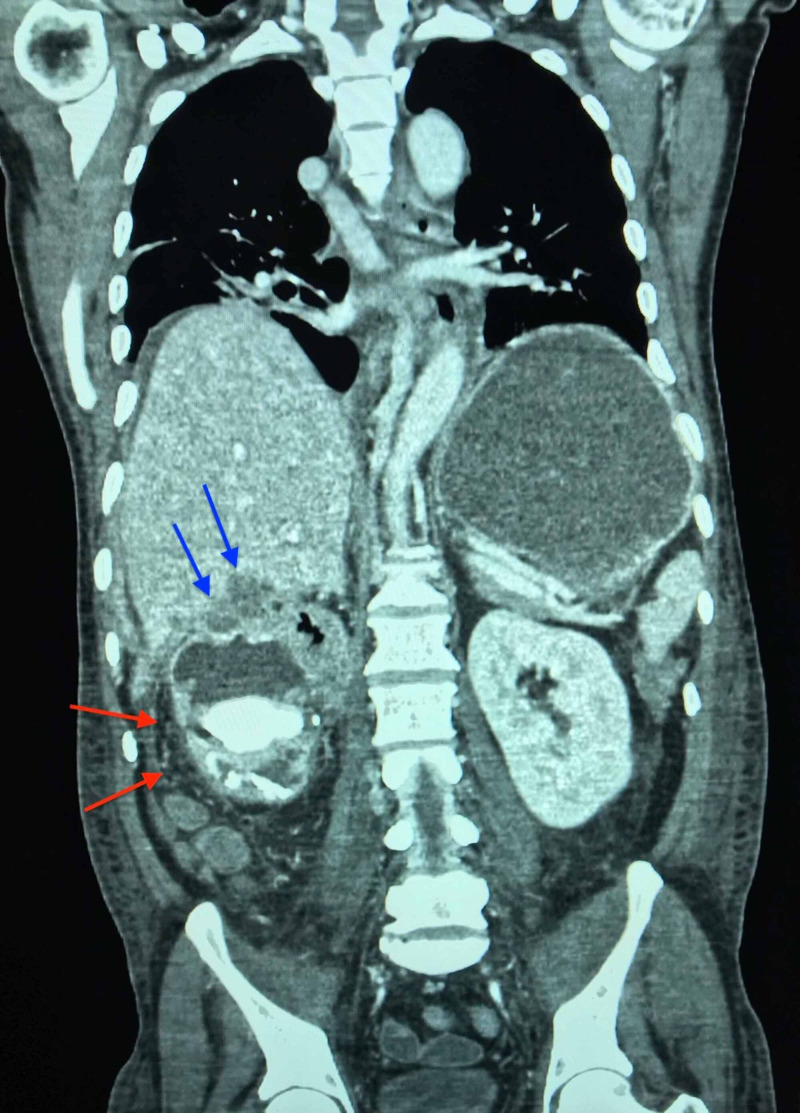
Subscapular hepatic hypodensities and inferior lateral renal densities visualized on CT of abdomen, coronal plane Subscapular hepatic hypodensities are indicated by the blue arrows. Inferior lateral renal nodular densities are indicated by the red arrows along the renal fascia.
